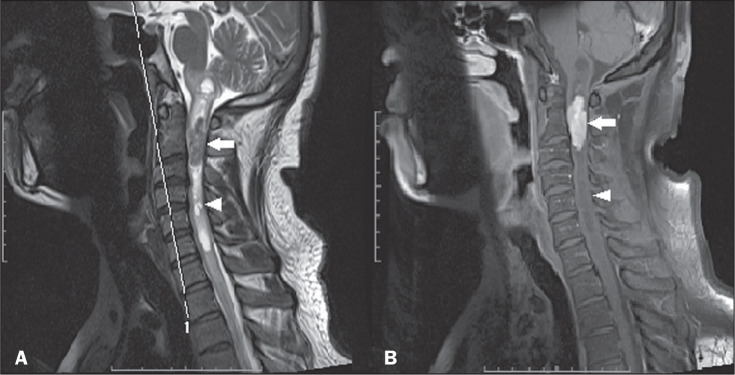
Ependymoma. Sagittal MRI scans. A: T2-weighted sequence showing an
